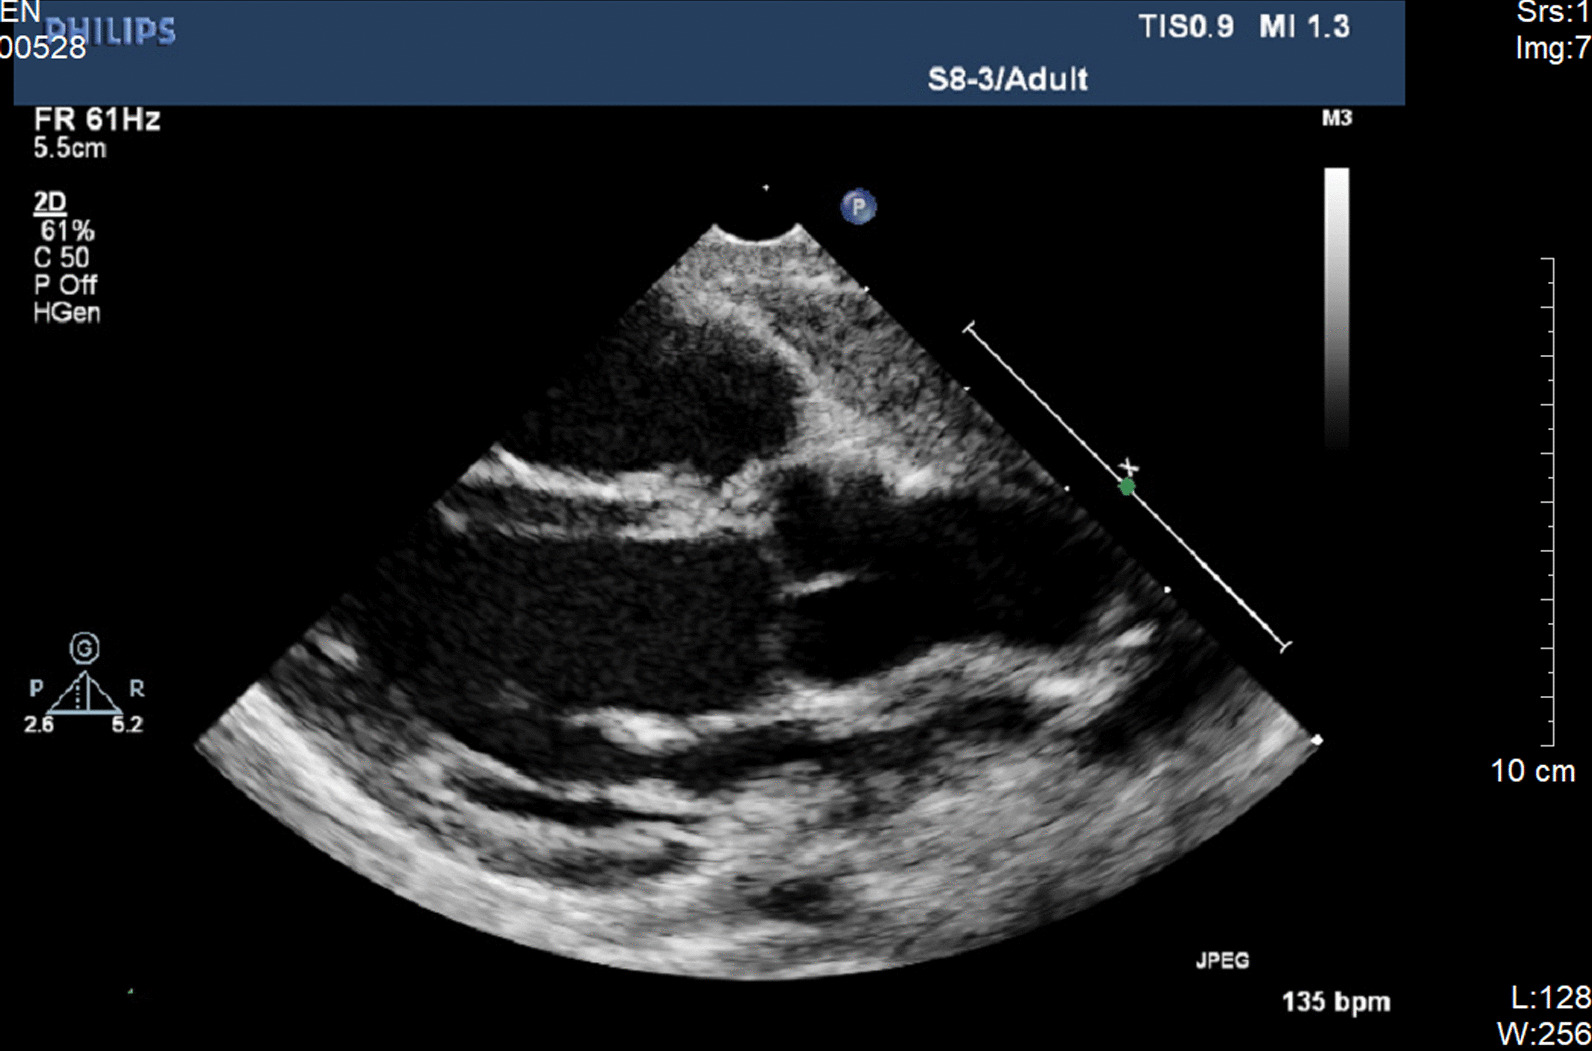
Echocardiography showing a broadened sinus of aorta
